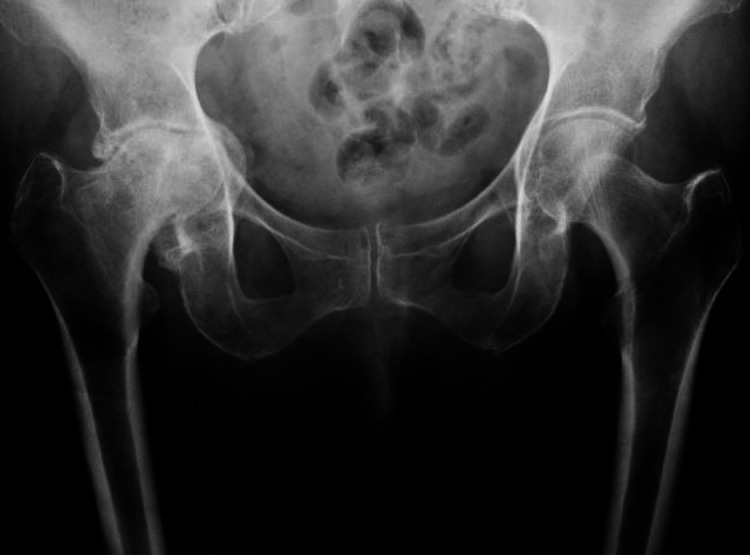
Anteroposterior radiograph of the pelvis showing characteristic protrusio acetabuli deformities. Note medial displacement of right femoral head past Kohler’s line, acetabular bone stock deficiency, incarcerated femoral head, and reduced hip offset and length.
